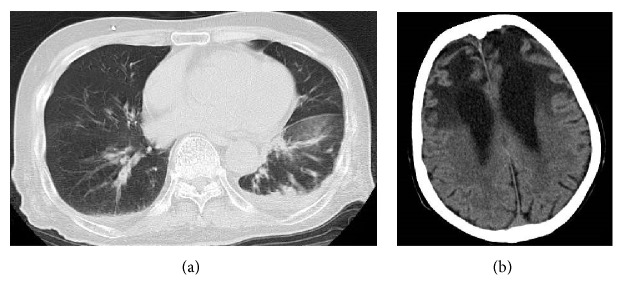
Plain nonenhanced computed tomographic images of the lung and head. (a) Image shows a consolidation suspected as pneumonia at the bottom of the patient's left lung with concurrent interlobar pleural effusion and pleural effusion. (b) Although acute-phase lesions such as bleeding were not seen, frontal lobe atrophy and ventricular enlargement were confirmed.
